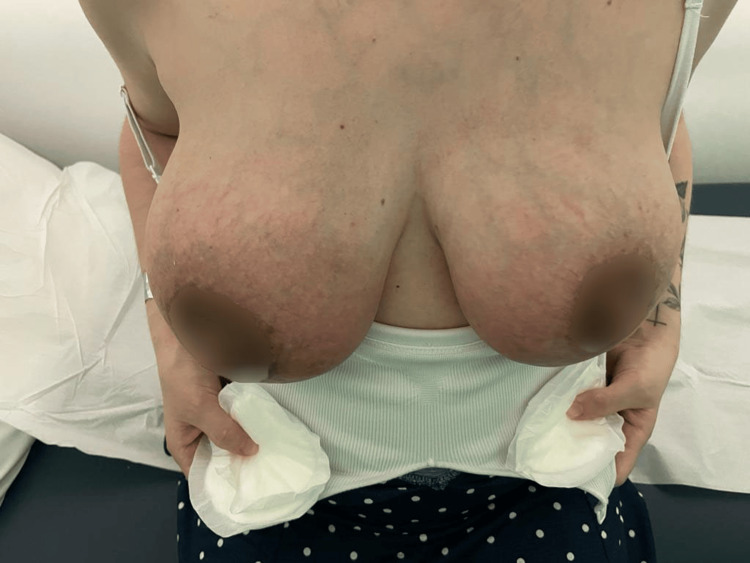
Day three of continuous therapeutic ultrasound
